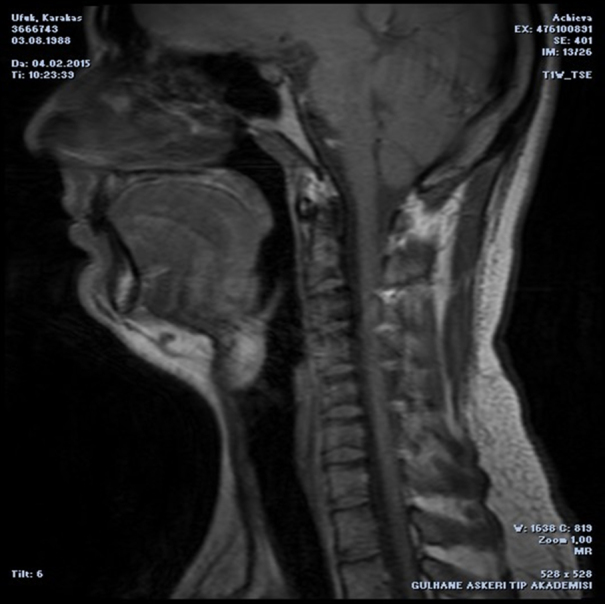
Postoperative sagittal T1-weighted MRI showed that the mass was totally resected.
